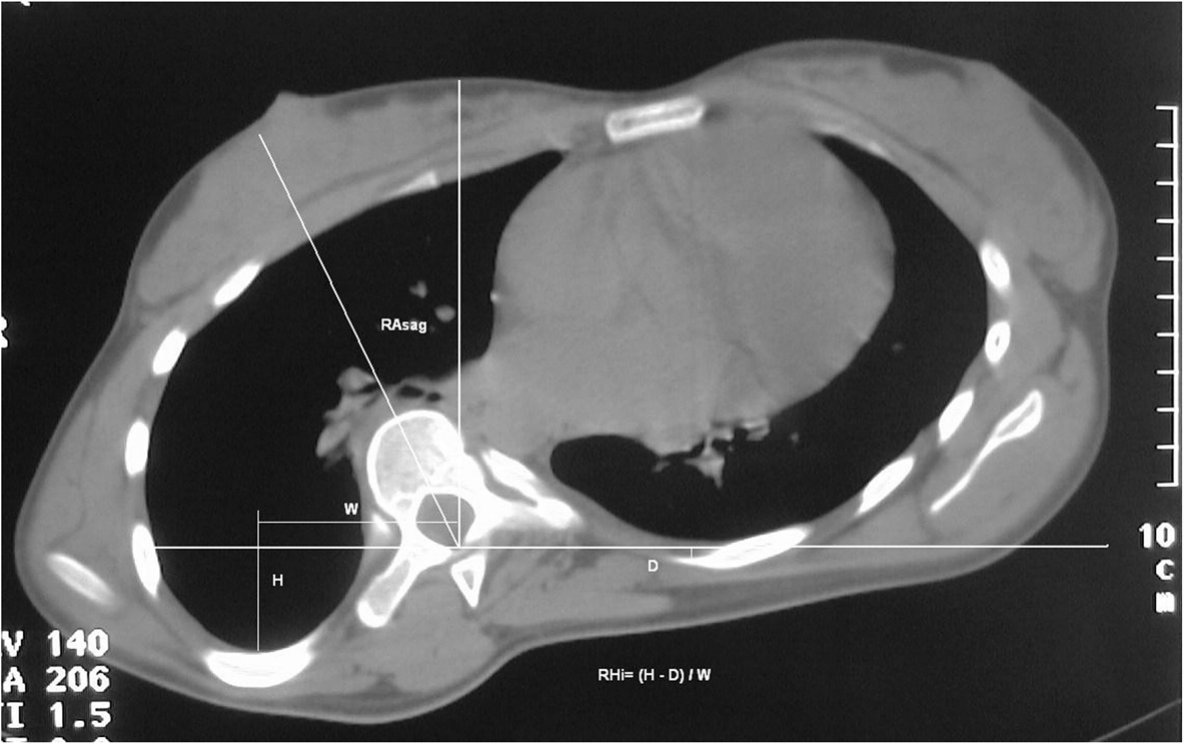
Spinal and rib cage CT scan at the apex of the curve. Measurement of the vertebral rotation angle about the longitudinal axis relative to the sagittal plane RAsag and Rib Hump index RHi defined as the ratio (H-D) / W.
